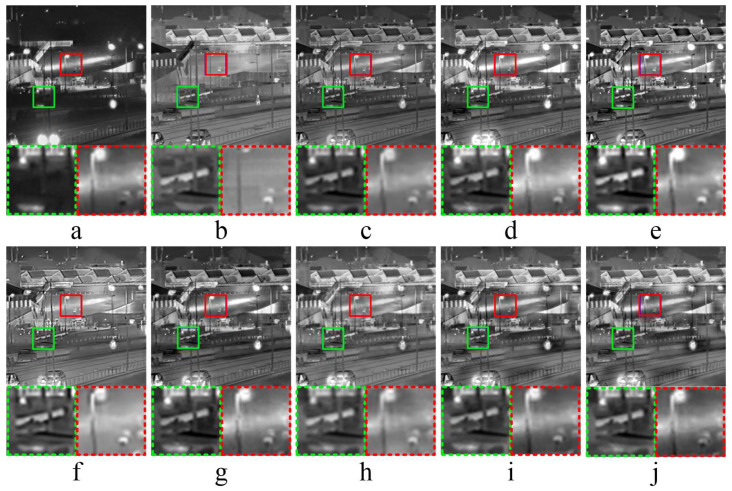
Infrared-visible Image Fusion Comparative Experiments—4. (a,b) are source images, (c–j) are the fused results of ASR, CNN, CT, KIM, MST-SR, NSCT-PCNN, NSCT-PCand the proposed method, respectively. At the bottom of each image, two areas marked in green and red dashed line frames correspond to the magnified areas encompassed in green and red frames, respectively.
